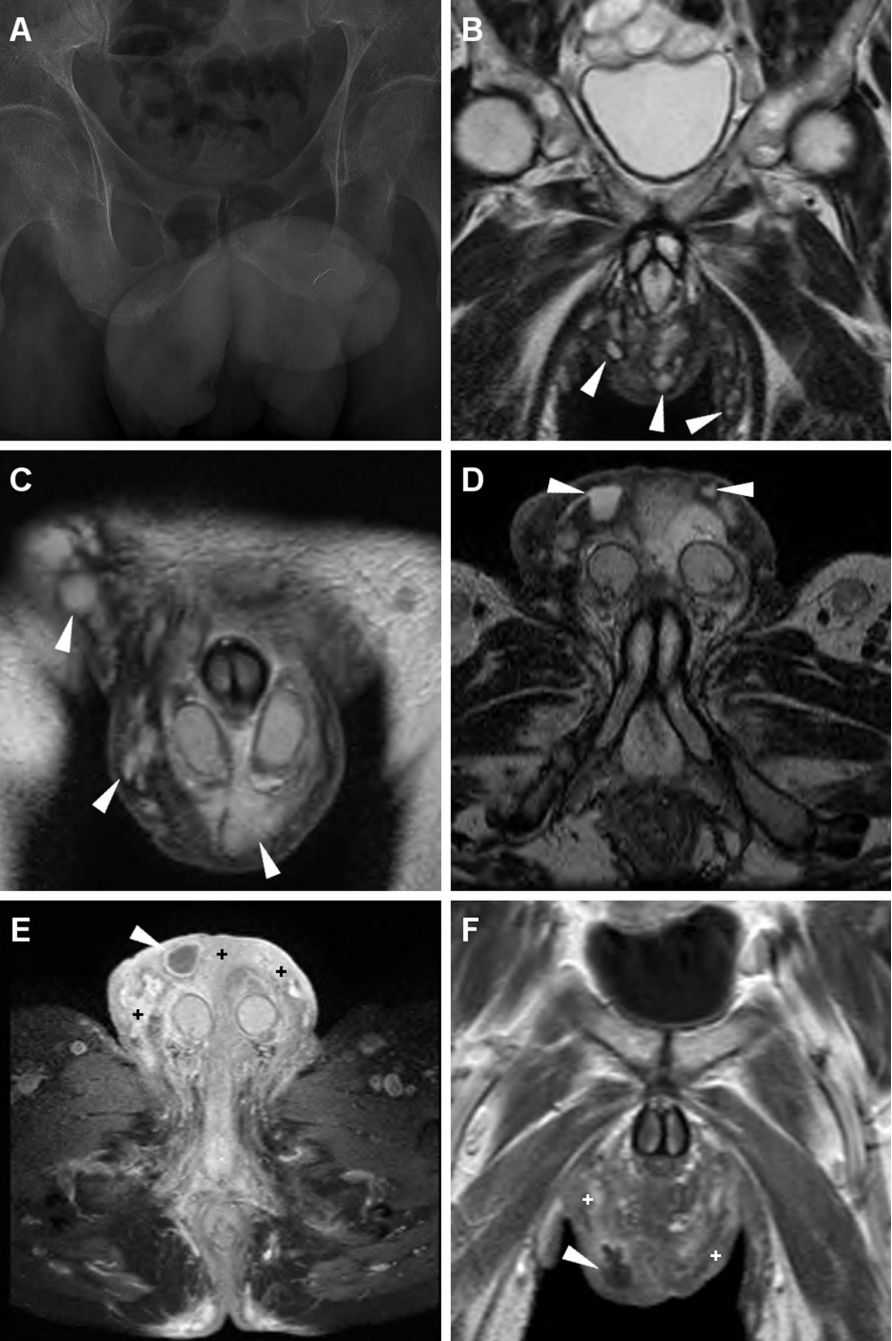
Surgically confirmed hidradenitis suppurativa in a 51-year-old man with hepatitis C, complaining of progressive, painful swelling of perineum, scrotum and penis, with thickened skin and fistulous orifices. Plain radiographs (a) excluded air collections in the swollen scrotum. MRI including multiplanar T2-(b–d), post- gadolinium axial fat-suppressed (e) and coronal (f) T1-weighted images depicted marked symmetrical thickening of the skin and subcutaneous planes with abnormal inflammatory signal intensity and hyperenhancement, (+ in e, f) involving the medial aspect of the thighs, perineal region and scrotum. Small purulent collections with peripheral enhancement (*arrowheads*) and inflamed inguinal lymph nodes were present. The testes (not shown) did not show appreciable abnormalities [Partially reprinted with permission from Ref. [71]]
